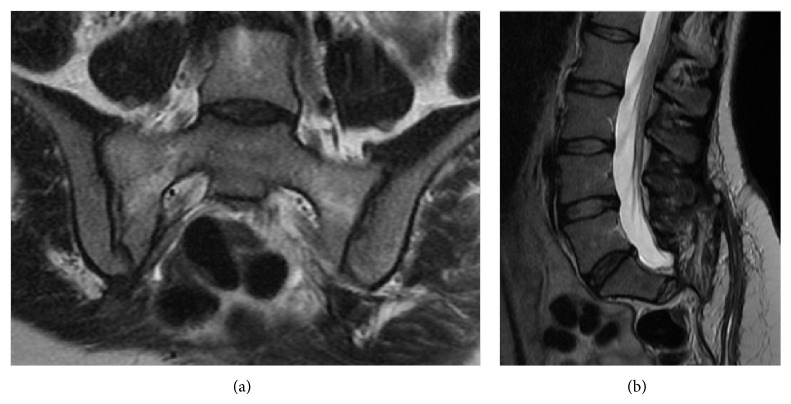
MRI scan evaluation 5 years after surgery, showing no recurrence of the tumor. (a) Coronal and (b) sagittal views.
